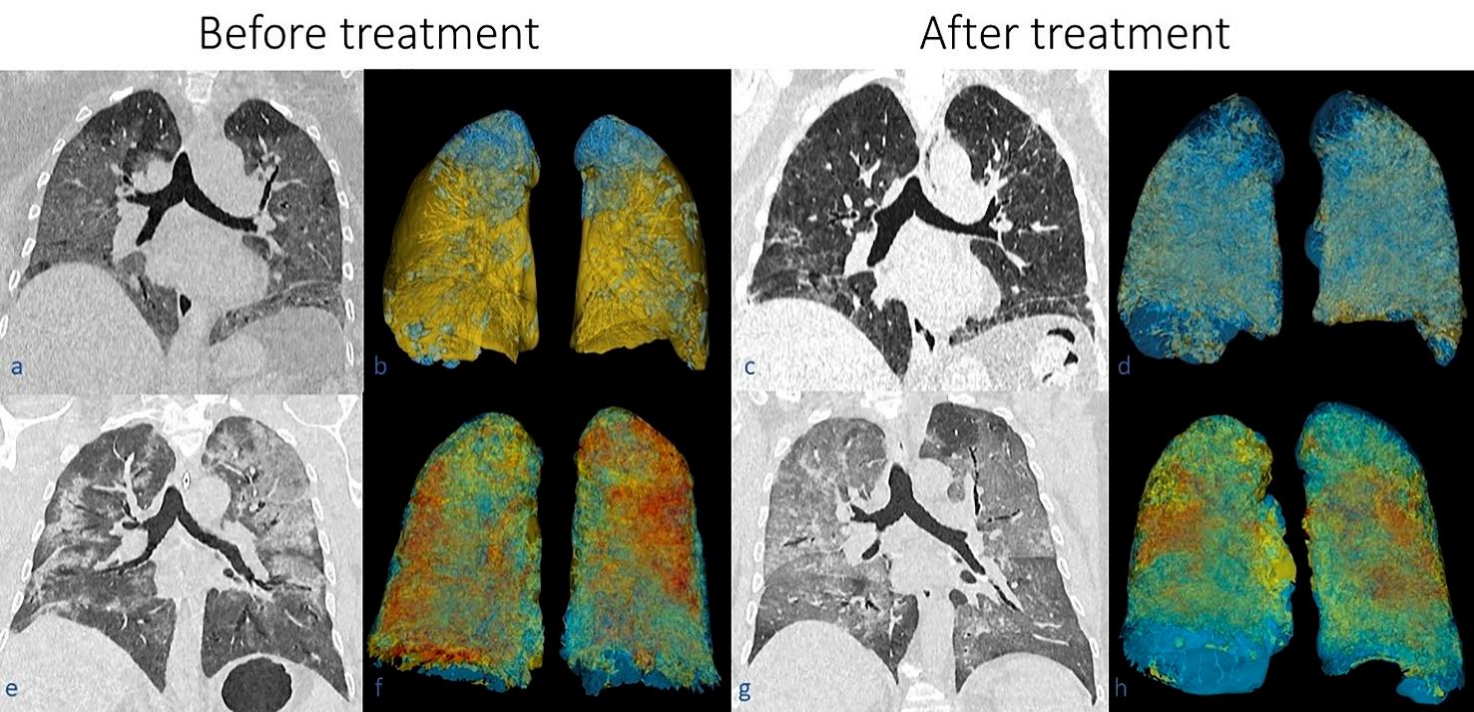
The images of the first row correspond to patient 1 (a, b, c, d), while images of the second row correspond to patient 4 (e, f, g, and h). CT scans in coronal plane are shown before (a, e) and after treatment (c, g) with their respective three-dimensional segmentation (b, and f before treatment; c and g after treatment). Notice in the affected lung parenchyma in the CT scans as ground-glass opacities and consolidations. In the three-dimensional lung segmentation, blue corresponds to undamaged parenchyma, the yellow color corresponds to ground-glass opacities, while the red color corresponds to consolidation. Notice the reduction of ground-glass opacities and consolidations in the lungs of both patients after treatment.
